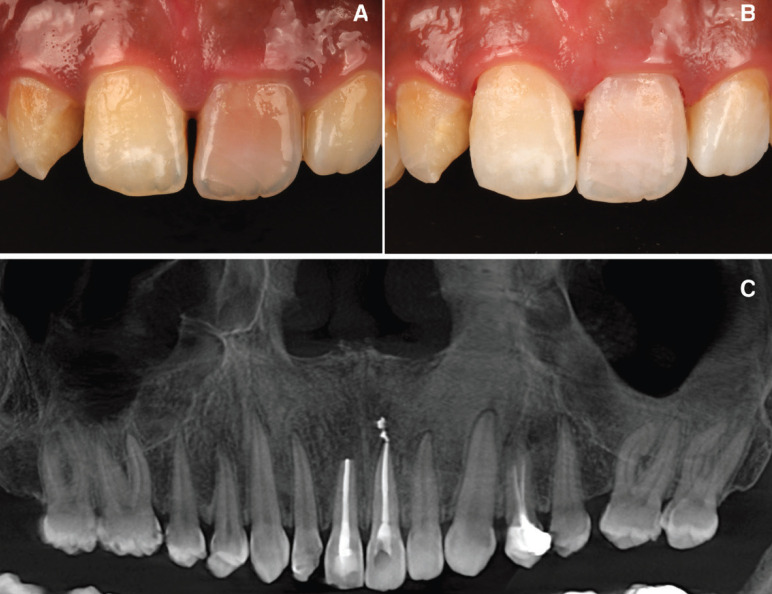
A) Contralateral tooth crown discoloration due to pulp necrosis. B) Contralateral crown bleaching due to crown discoloration after a pulp necrosis. C) Post treatment orthopantomography.
